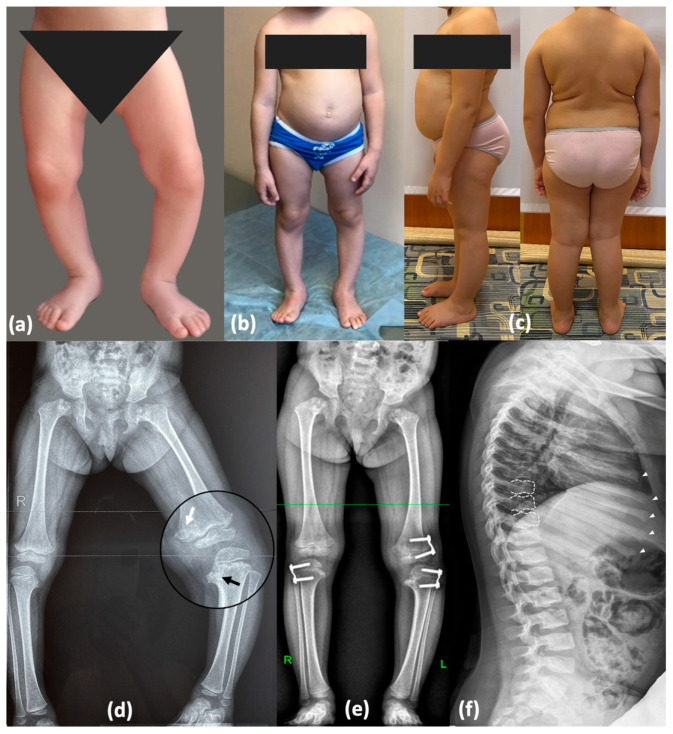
Phenotype and skeletal radiographies of P1 at various ages: Photos taken (a) at 1.5 years (before surgery); (b) at 3 years 7 months—after temporary hemiepiphysiodesis; (c) at 7 years 6 months—significant improvement was observed following plate removal and axial correction after 1.5 years. (d) Varus deformity of the lower limbs (3 y.o., before surgery)—severe on the left side and mild on the right side. Metaphyseal irregularities predominantly in the medial parts of the femur and tibia with mild “chondromatous” changes in the femur (white arrow) and Blount-like tibia vara (black arrow). (e) Correction of the deformities with the “guided growth” technique (3 years 7 months). Perthes-like fragmentation of the capital femoral epiphysis and widening of the femoral neck. (f) Irregularities of the vertebral endplates (white dotted lines) and enlarged distal parts of the ribs with rickets-like edges (white arrowheads).
